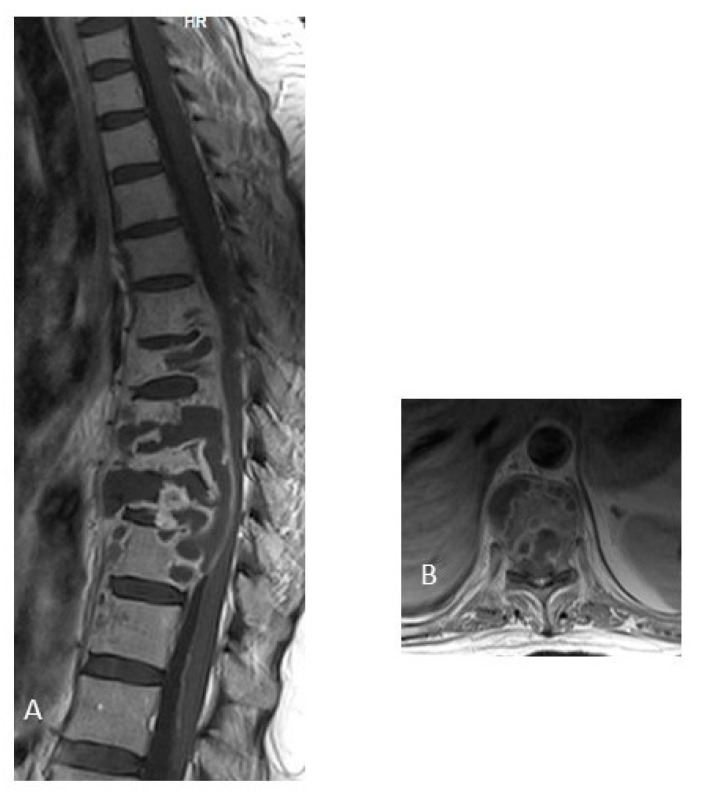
MRI of thoracic spine post-contrast T1-weighted image. (A) Sagittal image, and (B) Axial image, with lytic lesions of the vertebral bodies and appendiceal involvement from T7 to T9, with prevertebral and epidural abscess. A 29-year-old woman, with pulmonary tuberculosis, who had low back pain for 14 months, spinal tissue culture positive for *M. tuberculosis*. The image shows multilevel tuberculous spondylodiscitis from T5 to T11 with disc-vertebral destruction with epidural abscess with compression of the spinal cord from T7 to T10. (The photo is from our image gallery).
